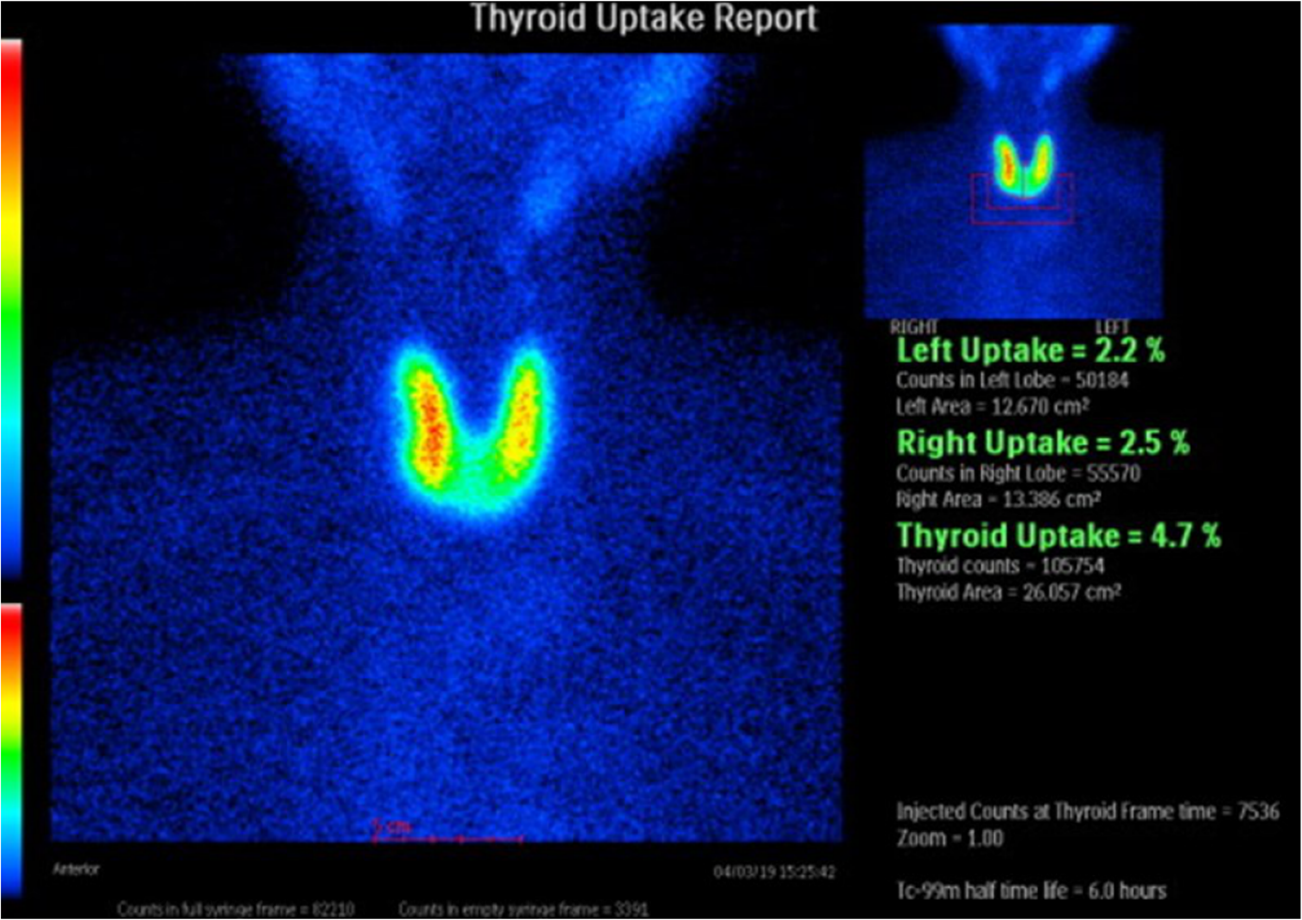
^99m^Tc-pertechnetate scintigraphy showing elevated, bilateral, and diffuse uptake of the radioactive tracer (4.7%)
